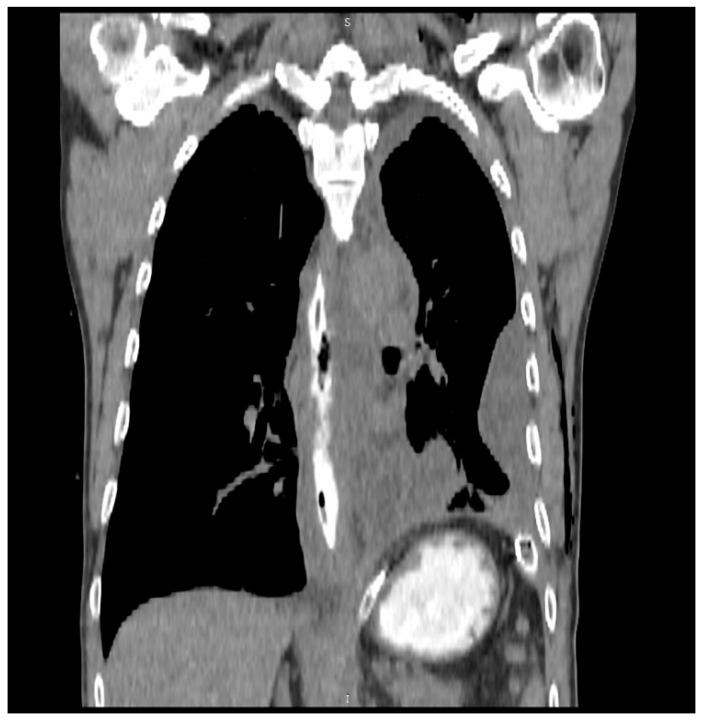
Follow-up longitudinal CT performed 10 days after surgery revealed favorable postoperative evolution. The esophagus appeared intact, without evidence of fistulous tracts, and there were no signs of gastroesophageal reflux or hiatal hernia. The cardia was patent, and the stomach maintained a normal tone and active peristalsis, with supple, well-preserved mucosal folds.
